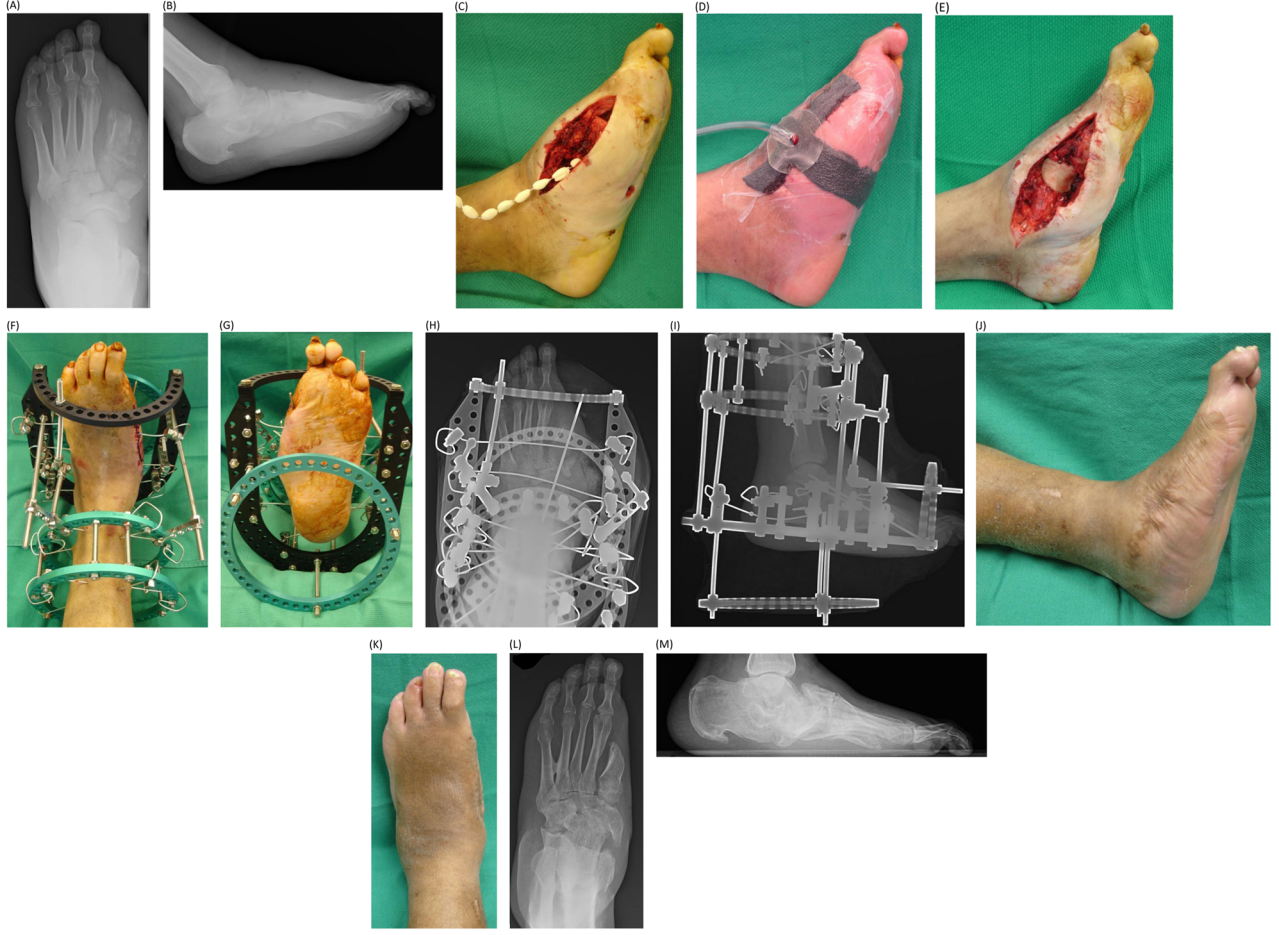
Pre-operative anteroposterior (A) and lateral (B) radiographic views showing the initial presentation of a diabetic Charcot foot with abscess and osteomyelitis. Patient underwent an initial surgical debridement and bone biopsy that was followed by revisional osseous and soft tissue debridement, application of non-biodegradable cemented antibiotic beads and negative-pressure wound therapy 2 days after the initial operation (C, D). The negative-pressure wound therapy was discontinued on post-operative day 6 followed by local wound care dressing changes. At approximately 10 weeks, the patient underwent removal of the non-biodegradable cemented antibiotic beads that was followed by a medial column arthrodesis, allogenic bone grafting and application of a circular, external fixation device for compression and surgical off-loading (E–I). The external fixator was removed at approximately 6 weeks that was followed by local wound care, further lower extremity casting, immobilization, and weight-bearing status with accommodative bracing. Final clinical (J, K) and radiographic (L, M) views at approximately 23 months follow-up.
